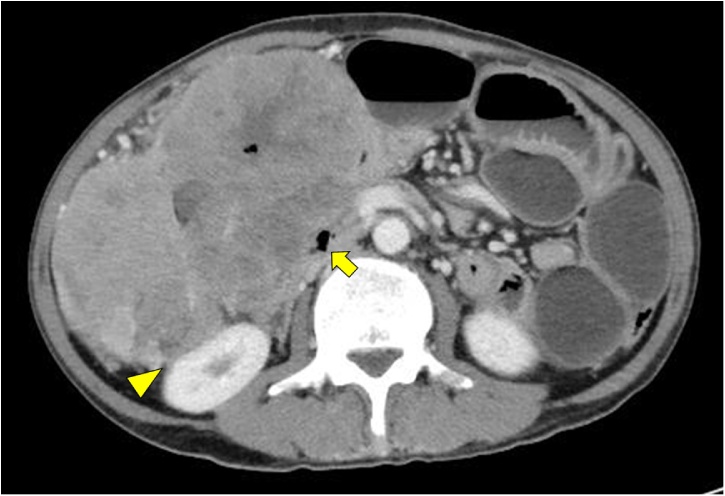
Abdominal CT on admission. Abdominal CT shows a huge, 18-cm mass in the right upper abdomen (Fig. 1). The tumor is located very close to surrounding organs such as the duodenum (arrow) and right kidney (arrow head).
